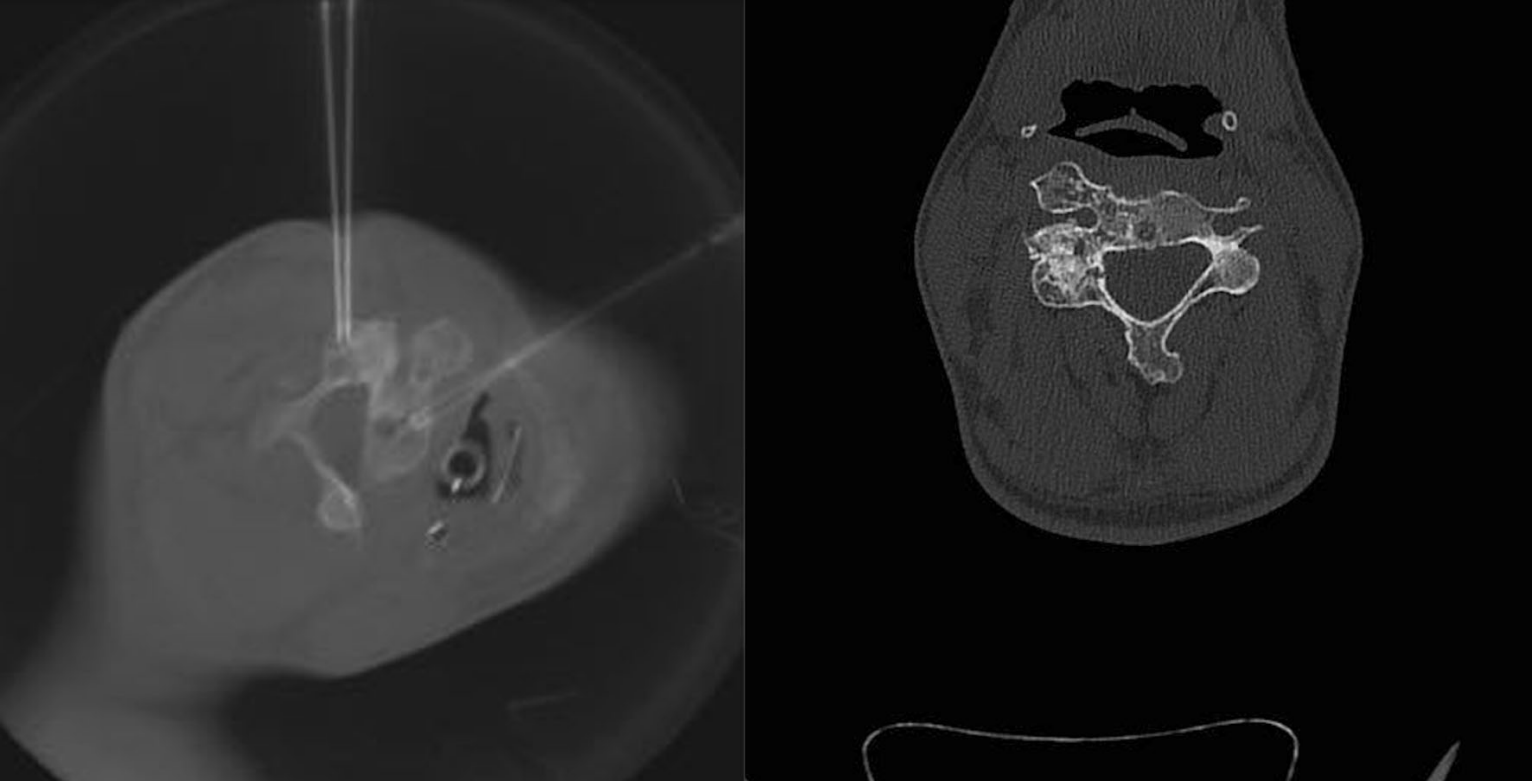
Needle placement inside the cystic chambers during the second sclerotherapy session (left) and CT image one year after the procedure depicting almost complete sclerosis within the cystic chambers (right)
